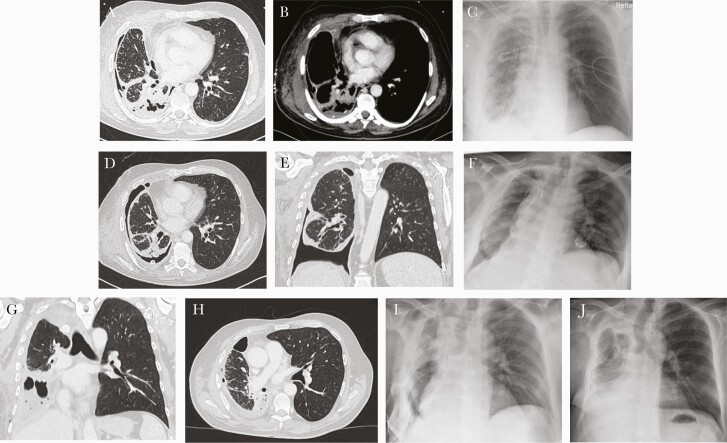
Initial computed tomography scan (a and b) and postoperative x-ray (c) after rethoracotomy and pleurectomy/decortications. Computed tomography scan 4 weeks later with persistent bronchopleural fistula and re-empyma (d and e) + postoperative x-ray after right lower lobectomy (f). Computed tomography scan another 4 weeks later with re-empyema and bronchial stump insufficiency (g and h) and postoperative x-ray after middle lobe lobectomy + latissimus dorsi flap (i). Final x-ray after discharge 3 weeks later (j).
